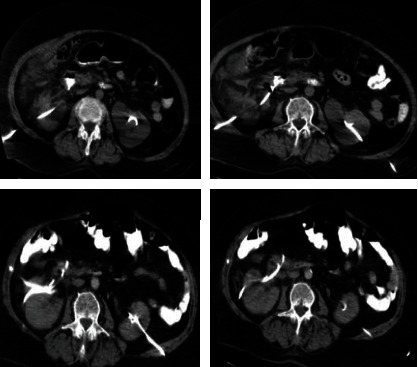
Oral and intravenous contrast-enhanced abdominal CT images. The right nephrostomy tube was located in the second part of the duodenum, and there was no leakage of contrast substance to the retroperitoneal site.
